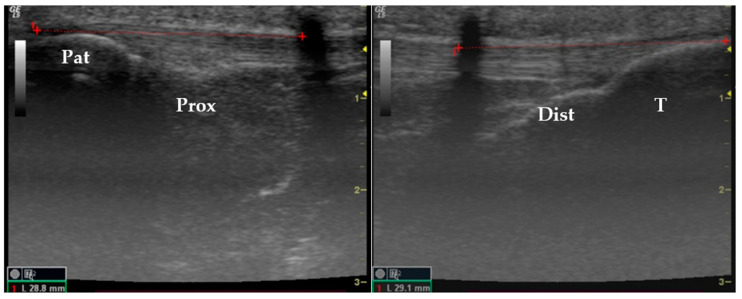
Longitudinal section of an ultrasound image showing the length measurement of the patellar tendon. Pat: patella; T: tibia; Prox: proximal; Dist: distal.
